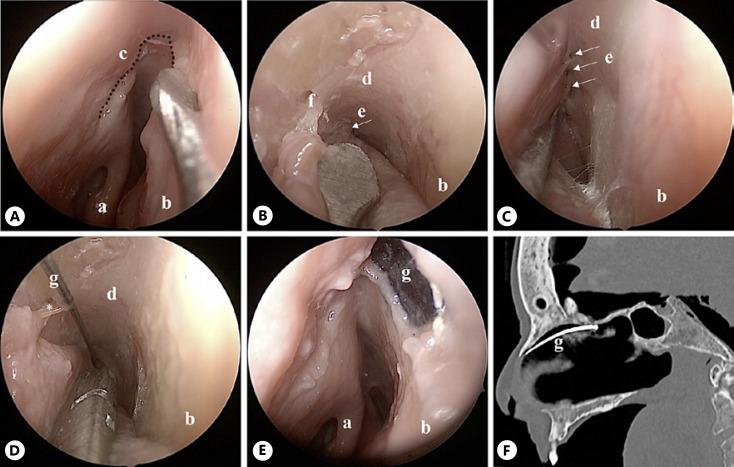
Endoscopic intranasal positioning of the electrode (approach 1), right nasal fossa. A Harvesting of the U-shaped mucosal flap. B, C Preparing the olfactory cleft as posterior as possible. D The electrode is placed in the olfactory cleft. E The mucosal flap is replaced to cover the electrode so that only a sensor attached to the electrode is seen on endonasal mucosa. F Sagittal CT scan showing a part of the intranasal positioning of the electrode. a, middle turbinate; b, nasal septum; c, dotted line, mucosal incision for the U-shaped flap; d, olfactory cleft; e, fila olfactoria; f, septal branch of anterior ethmoidal artery; g, electrode.
